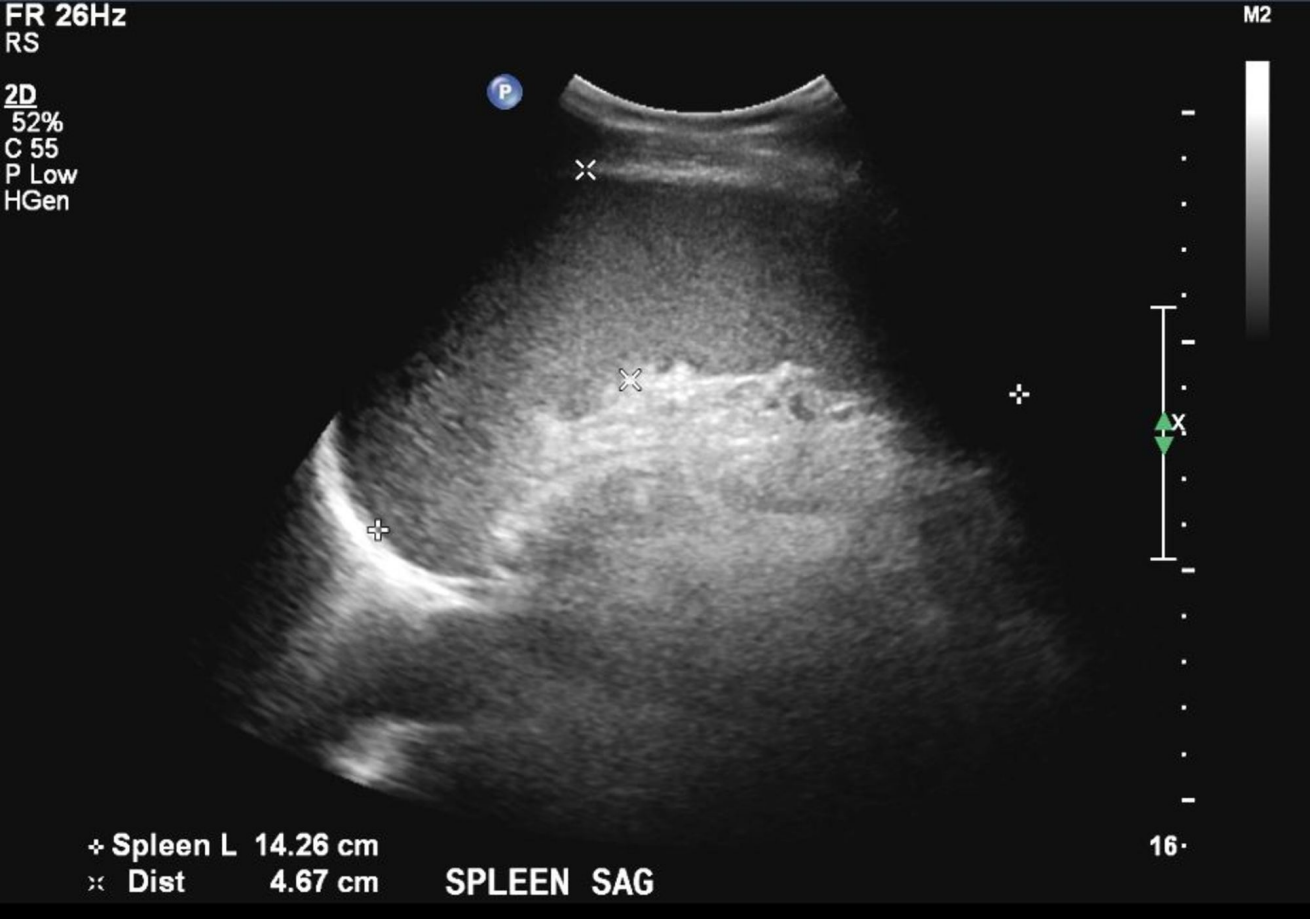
Abdominal ultrasound showing mild splenomegaly.
